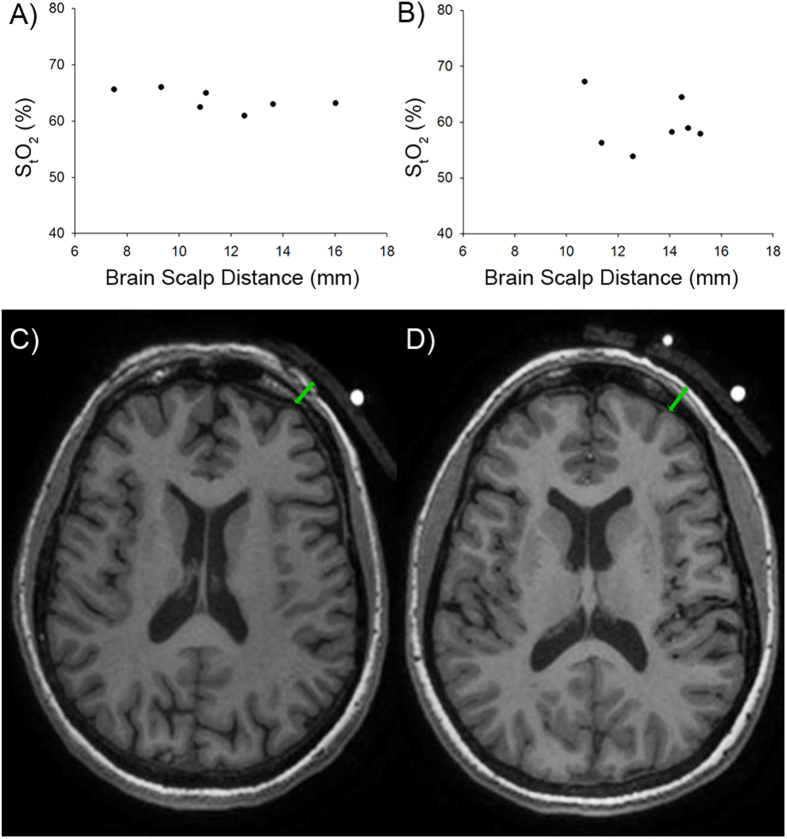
Combined MRI/NIRS measurement. There was no significant relationship between S_t_O_2_ and brain scalp distance in controls (A) and MS patients (B). Representative images of a control and MS patient are shown in panel C and D, respectively. Bright dot represents the location of the Vitamin E capsule placed besides the source and detector.
